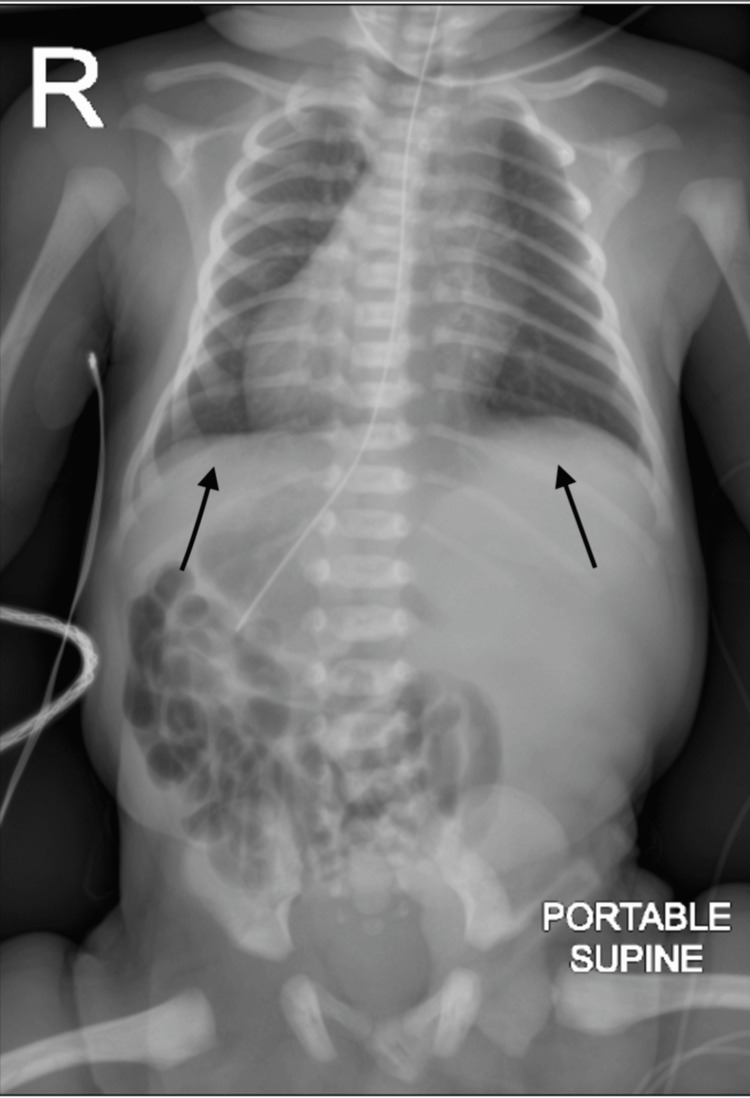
Chest X-ray Chest/Abdomen Radiograph revealing mild hyperinflation of both lungs with perihilar streakiness.
